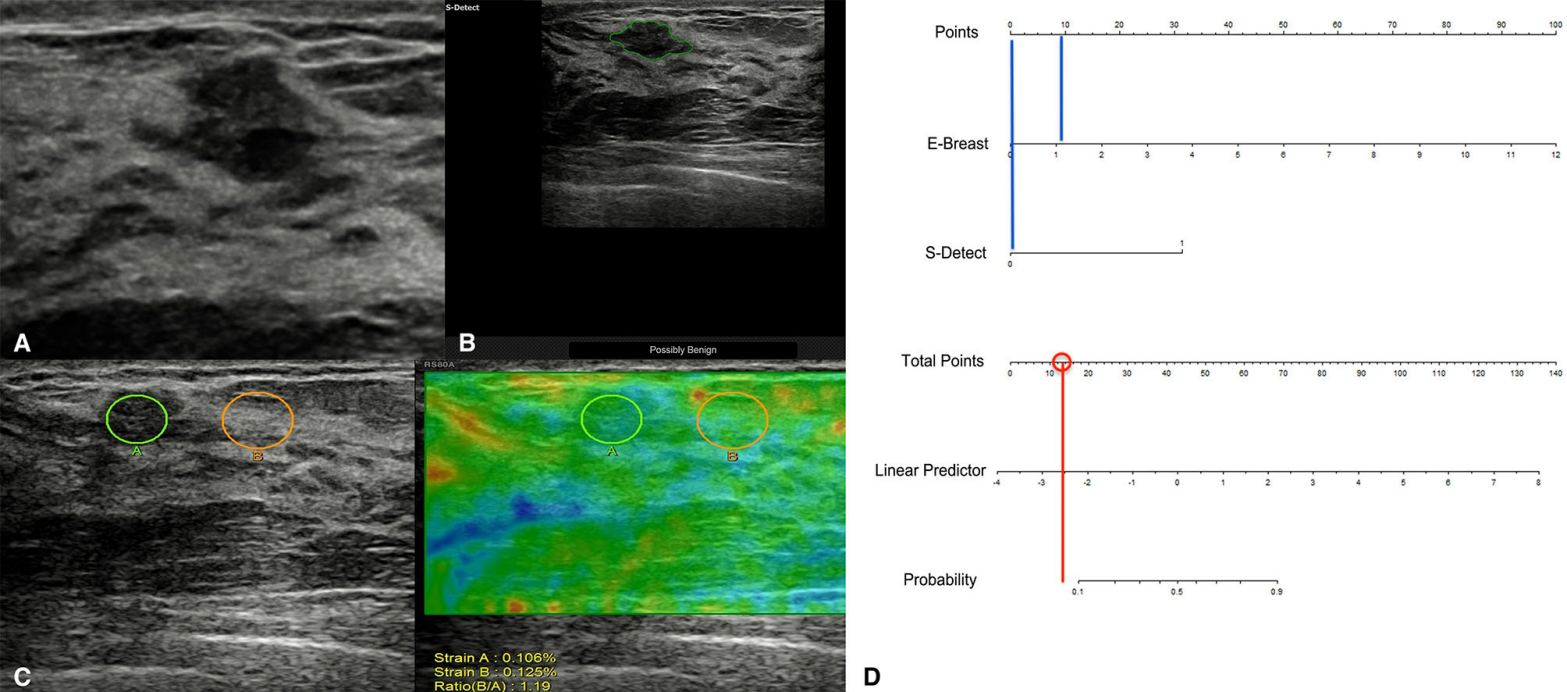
A typical case of a 45-year-old patient with a breast lesion detected and classified as BI-RADS 4a by screening ultrasound (US) (A; grayscale of the US). S-Detect classified it as possibly benign (B), and its strain ratio (SR) was 1.19 (C). According to the nomogram, the point for SR result was near 10, and the point for S-Detect was 0 (blue vertical lines), thus acquiring a total score of 10 for the lesion and a predictive percentage of less than 0.1 (D). The pathological result for the lesion was adenosis.
